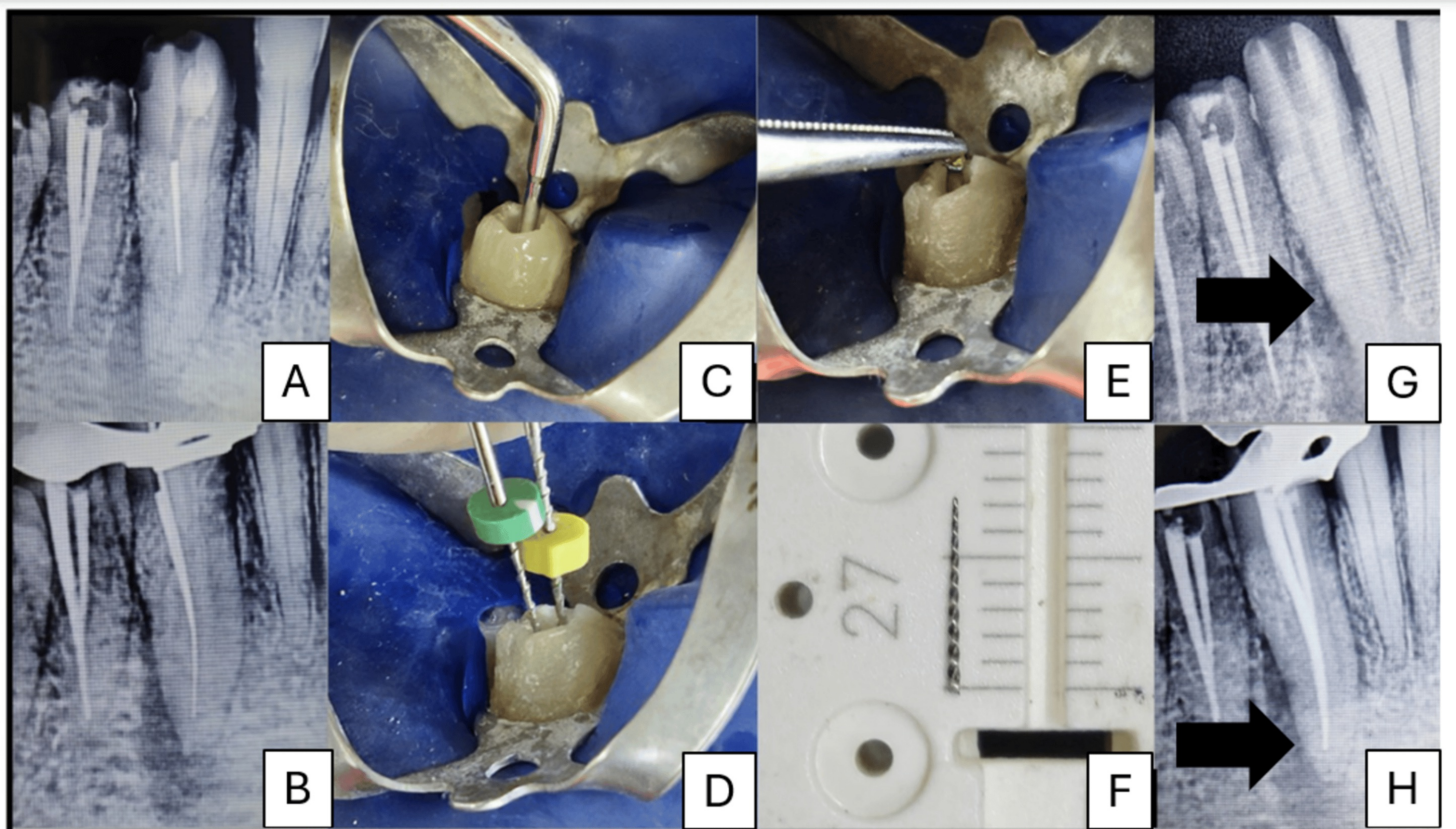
File retrieval from tooth 43 using ultrasonics and braiding technique. (A) Pre-op IOPA of 43 with a broken instrument. (B) Missed lingual canal negotiated. (C) Ultrasonic tip T3 is used to loosen the separated file. (D) Braided technique with H-files (#20, #35). (E) Instrument retrieved from the canal. (F) 7-mm-long retrieved file fragment. (G) Radiograph confirming complete file retrieval. (H) Post-obturation radiograph. IOPA: intraoral periapical
